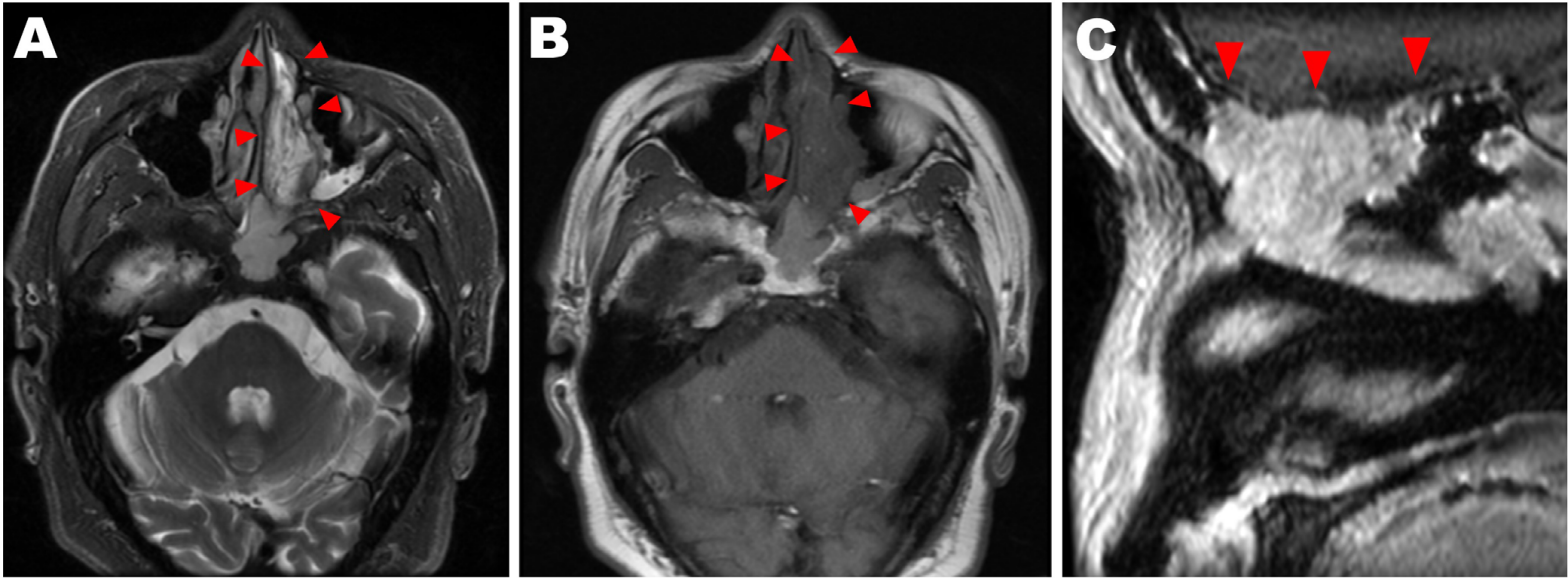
MRI at initial presentation. The lesion shows hyperintensity on T2‐weighted imaging (A), hypointensity on T1‐weighted imaging (B), and no extension beyond the anterior skull base to the intracranial space (C).
